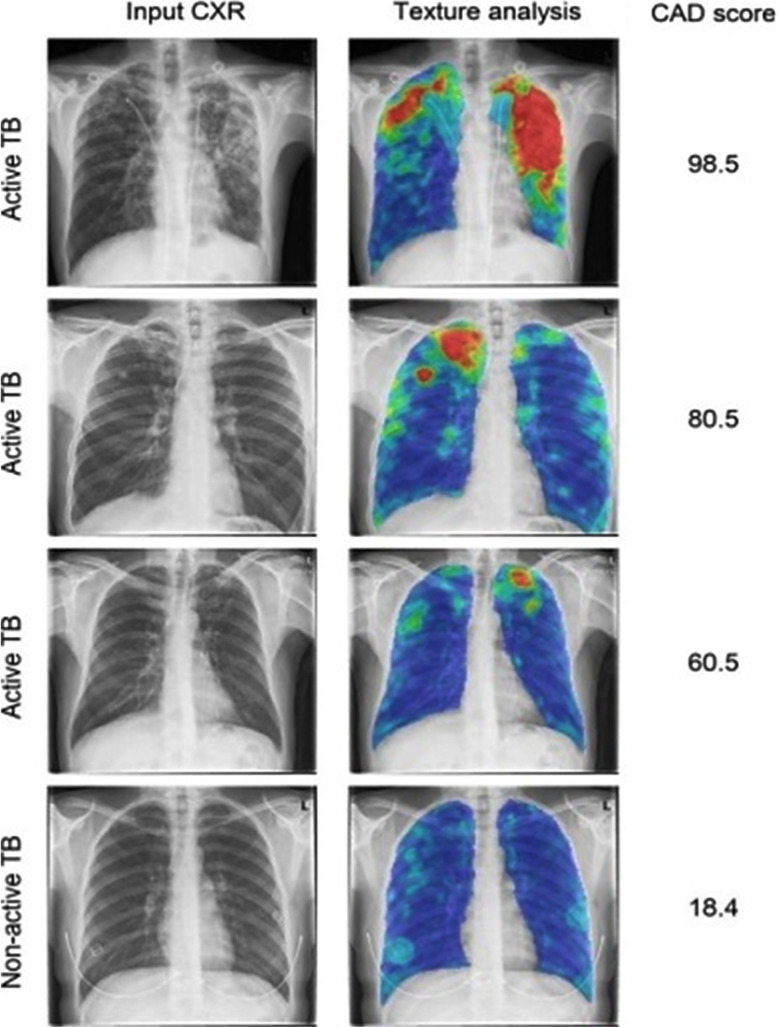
Pictorial Comparison of Input Chest Xray and CAD4TB Scores.
